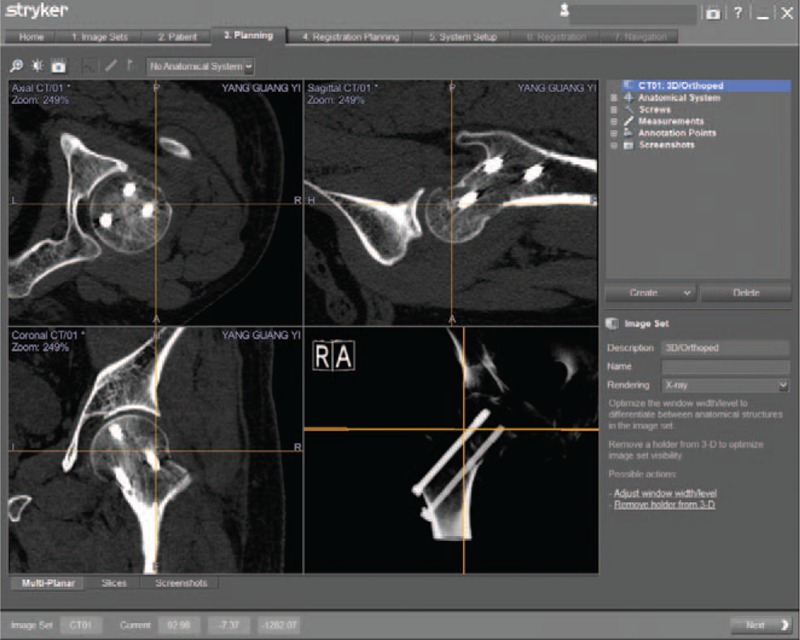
Postoperative CT of the right hip was scanned. Image data input into computer navigation showed that all the screws were in good position without penetrating the cortex. CT = computed tomography.
